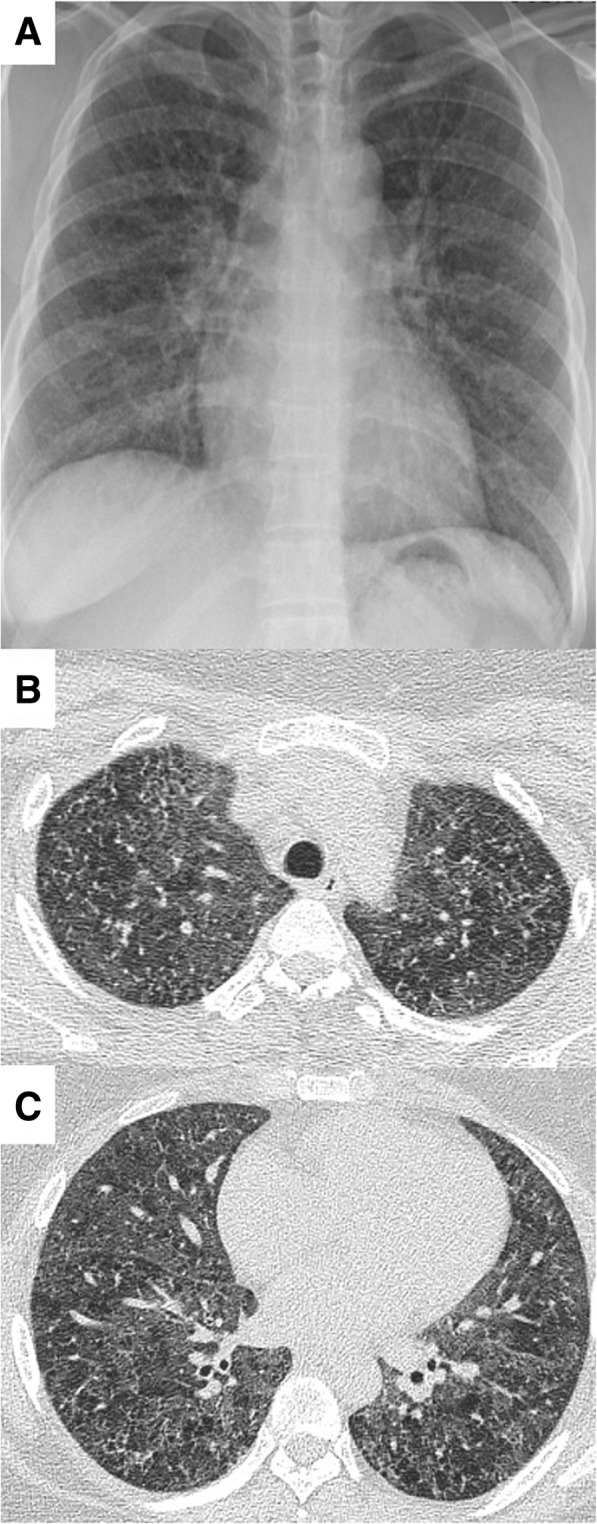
Imaging findings of the chest in the patient at hospital admission. a Chest X-ray showing diffusely reticulo-nodular densities in the whole lung field. b HRCT of the chest revealing central oriented ground glass opacities with some cystic lesions and airway trapping. c They had no lobar predominance with sparing subpleural area
